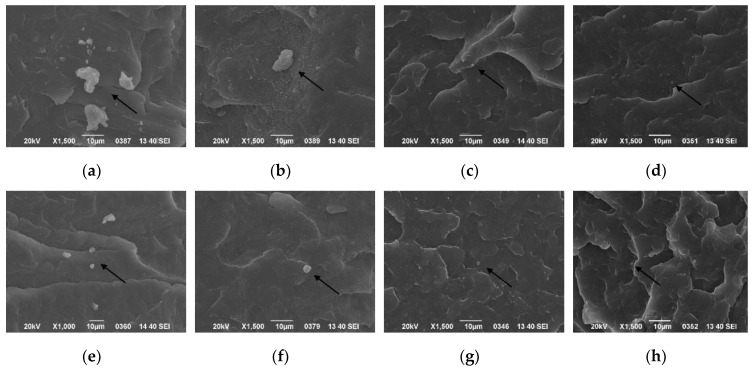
Dispersion of the nucleating agent under different cooling temperatures without ultrasound: (a) 30 °C, (b) 40 °C, (c) 50 °C, (d) 60 °C; and with ultrasound: (e) 30 °C, (f) 40 °C, (g) 50 °C, (h) 60 °C.
